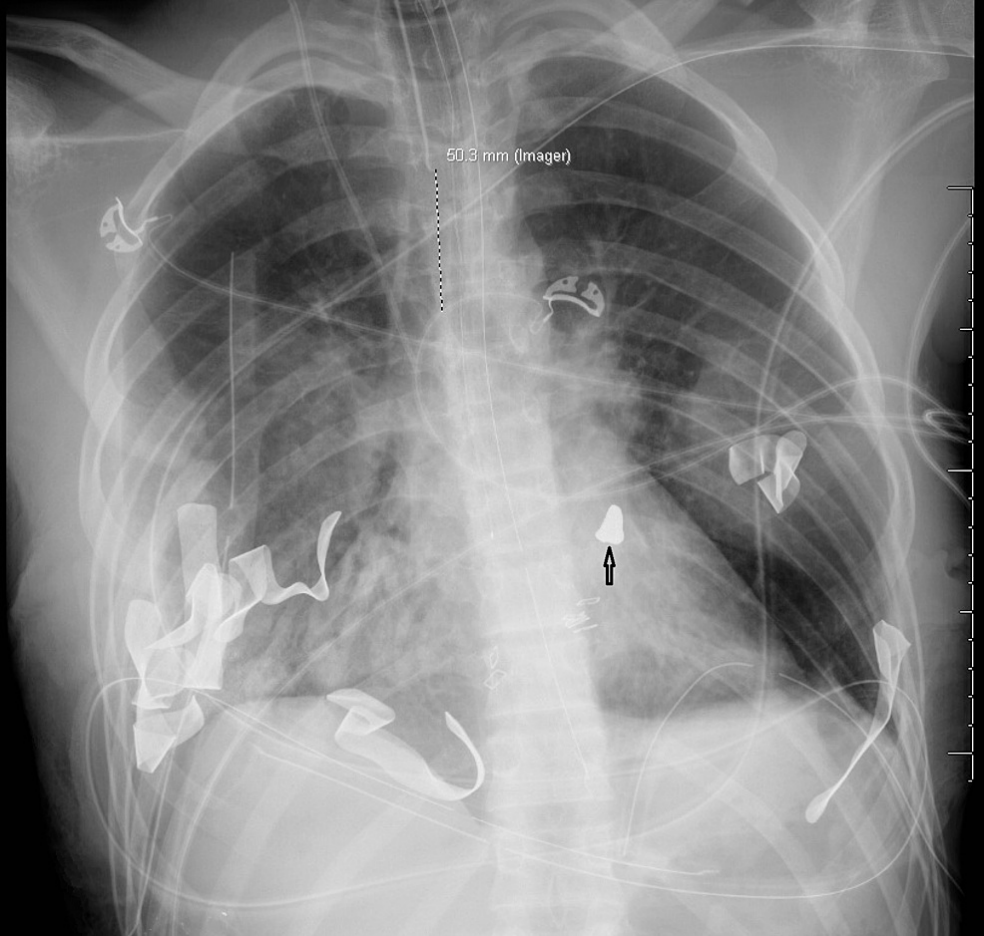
Chest X-ray (CXR) Obtained on Post-Operative Day 1 Figure 1 shows Chest X-ray (CXR) obtained on post-operative Day 1, revealing intrathoracic packing and missile (arrow) within the cardiac shadow.
